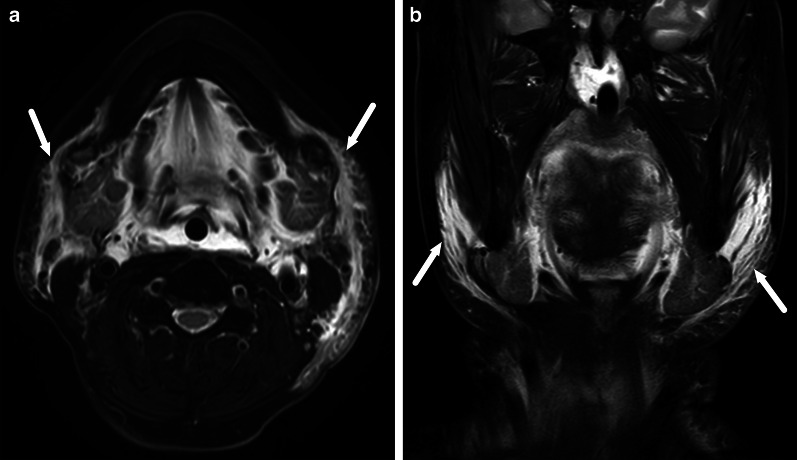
Patient with acute angioedema shows widespread edema in the neck soft tissues on axial (a) and coronal (b) fat-suppressed T2-weighted images (arrows), but no abscesses
